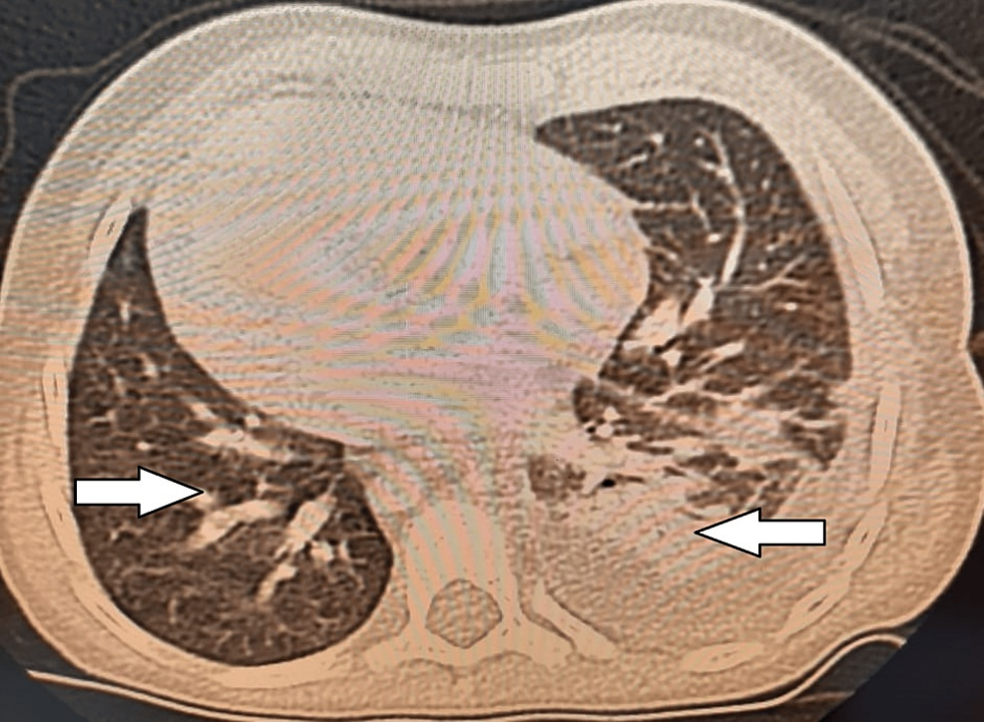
Thoracic CT scan (parenchymal window) showing situs inversus, bronchial dilatation (right arrow), and left laterobasal and posterobasal thoracic empyema (left arrow)
